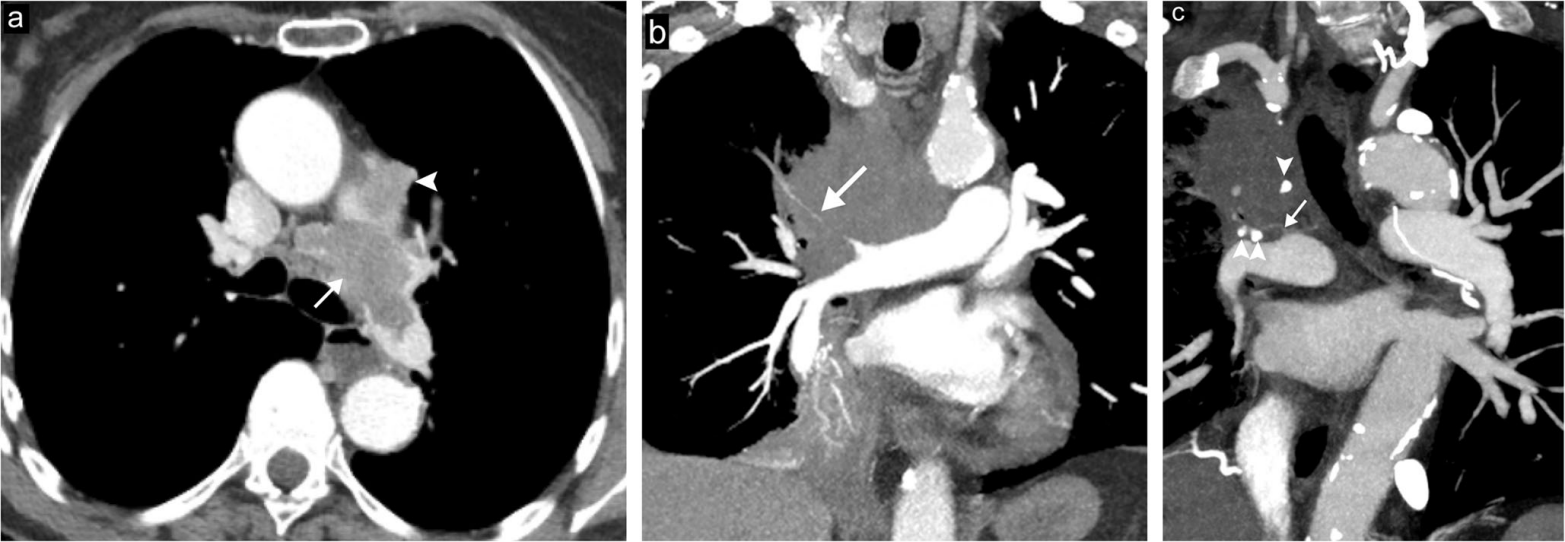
Pulmonary artery narrowing/obstruction. a Axial contrast-enhanced CT image shows a large, irregularly shaped filling defect within the left pulmonary artery (*arrow*) with extension into the mediastinal fat (*arrowhead*) causing intrinsic narrowing/obstruction of the pulmonary artery in a patient with biopsy proven angiosarcoma. b Coronal CT image demonstrating severe narrowing of the right upper lobe pulmonary artery (*arrow*) secondary to extrinsic mass effect from a right upper lobe primary lung malignancy. c Coronal CT image demonstrating complete occlusion of the right upper lobe pulmonary artery (*arrow*) secondary to extrinsic compression from biopsy proven fibrosing mediastinitis. Note the coarse calcifications present within the region of fibrosis (*arrowheads*)
